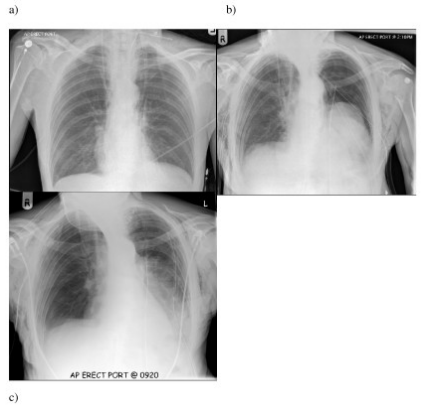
Series of chest radiographs. a) Chest radiograph in recovery showing bilateral pneumothoraces despite transhiatal chest drains. Note corrugated drain in left side of neck. b) Chest radiograph taken on day 1 revealing collapse of left lung despite chest drainage. Note resolution of right pneumothorax. c) Chest radiograph demonstrating full expansion of lung after removal of corrugated neck drain.
